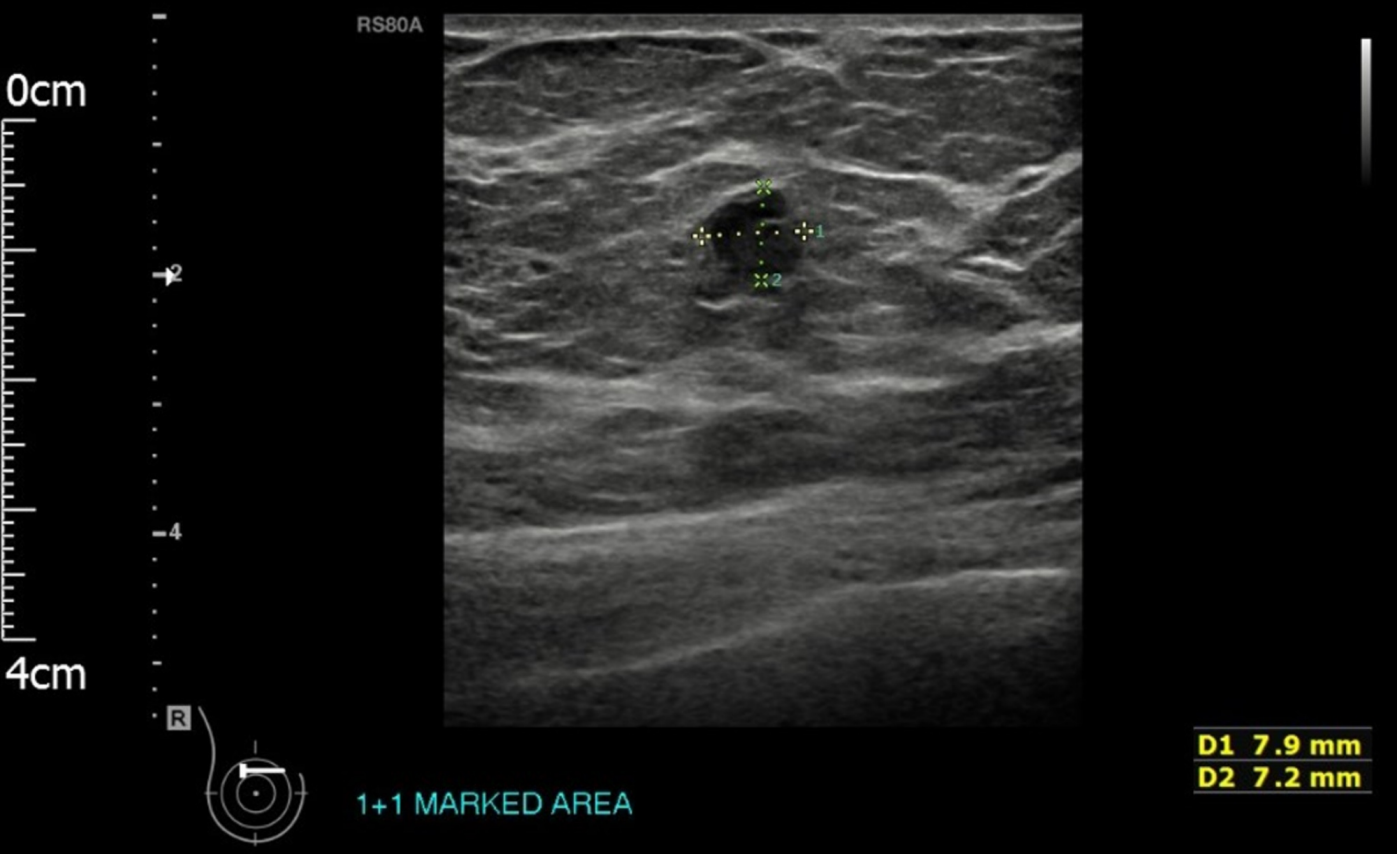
Right breast ultrasound showing the right breast lesion
